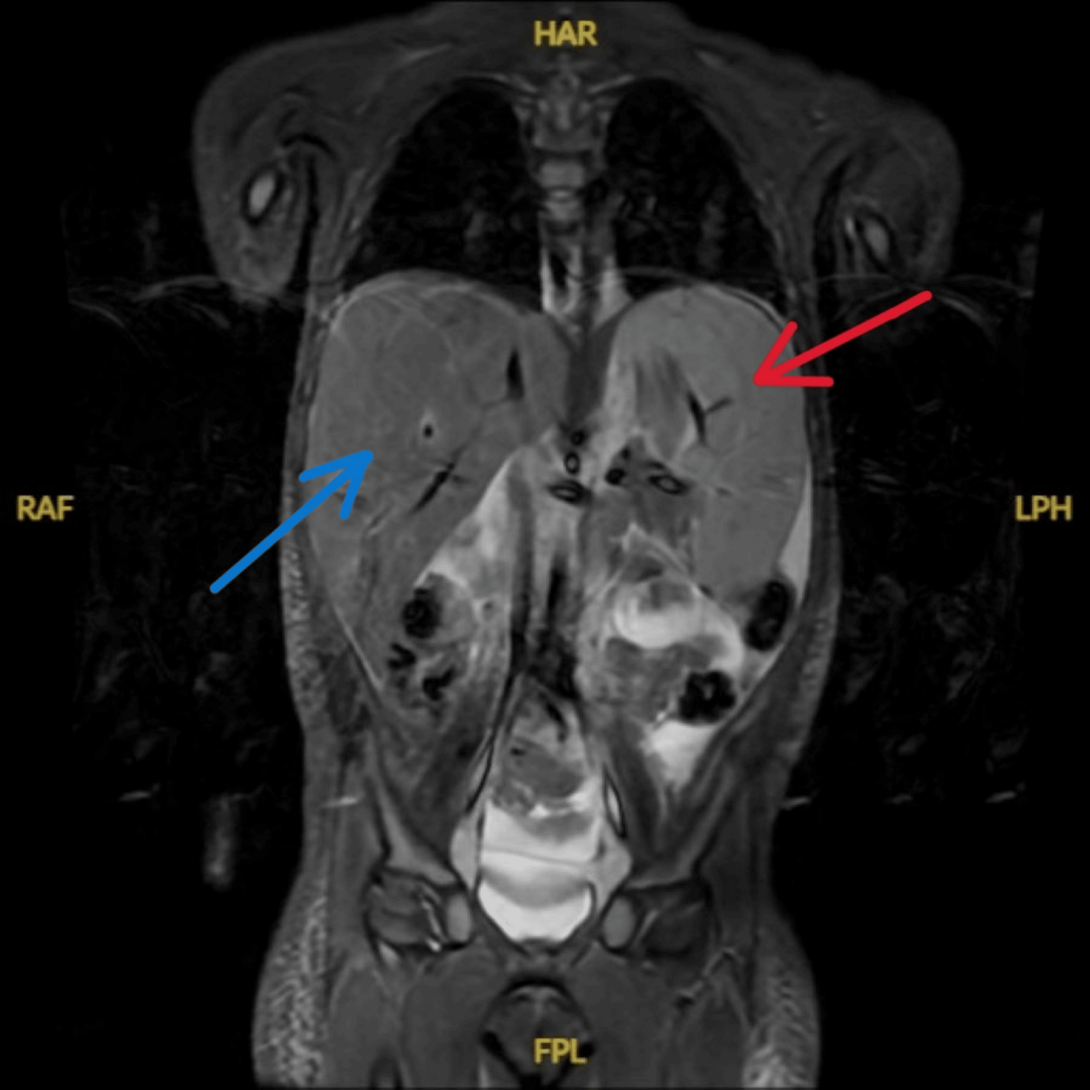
Whole-Body MRI (Coronal View) Demonstrating Massive Hepatomegaly (Blue Arrow) and Splenomegaly (Red Arrow) MRI, magnetic resonance imaging.
